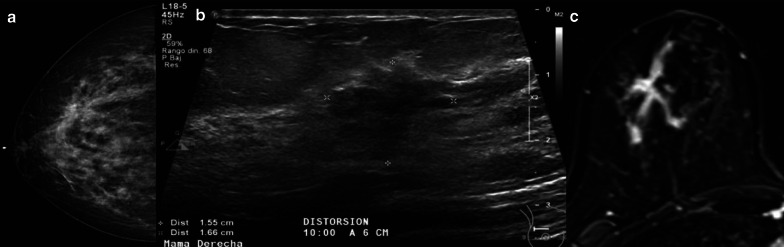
a Mammography (craniocaudal view) demonstrated hyperdense distortion in the upper outer quadrant. b Ultrasound shows a corresponding irregular and hypodense mass, 1.6 cm, at 10:00 o´clock. c The mass was confirmed on magnetic resonance imaging as a non-mass enhancing corresponding to classic lobular carcinoma in situ (10 cm) with microinvasion (< 1 mm)
